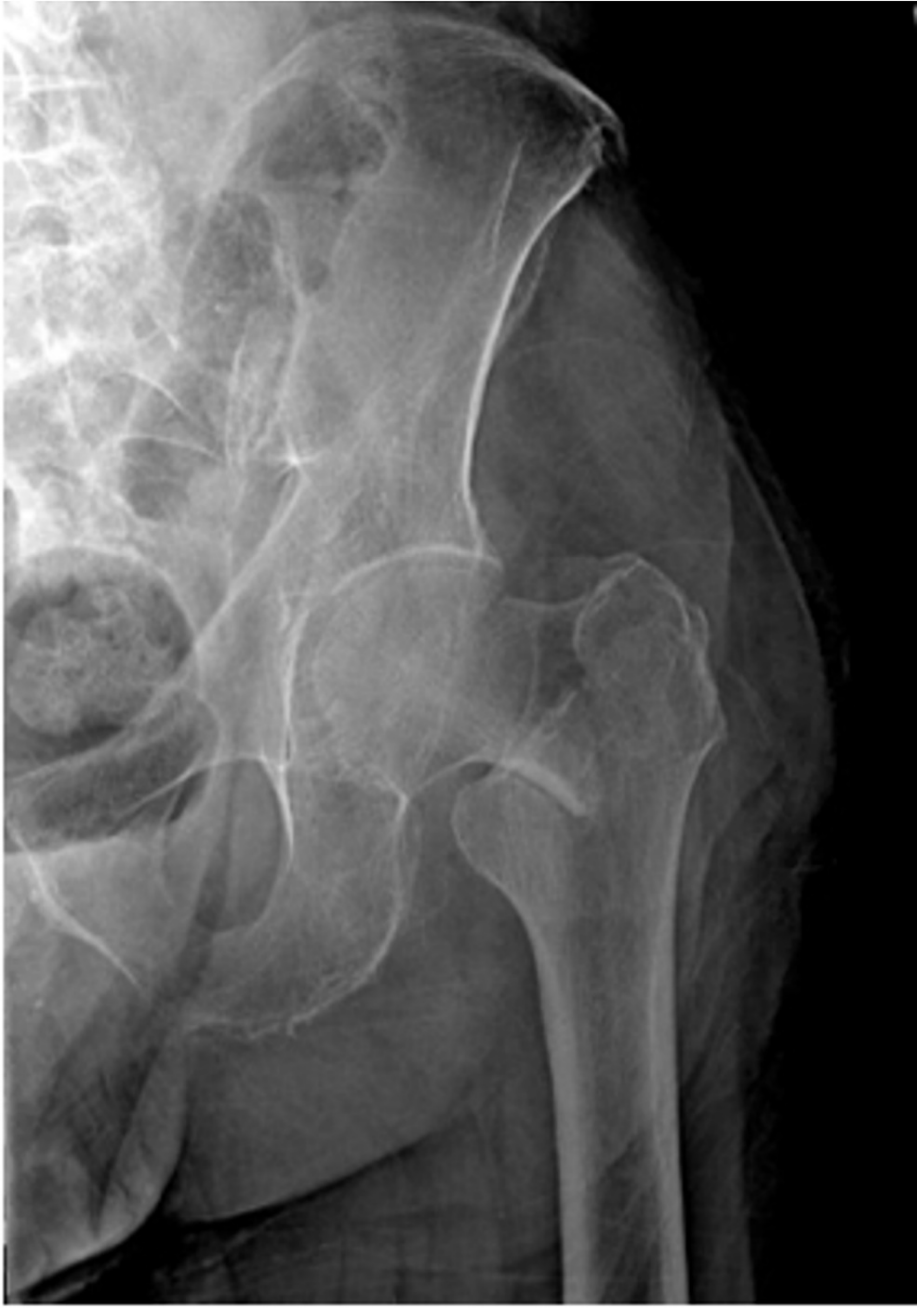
Radiograph demonstrating a basicervical femoral neck fracture
